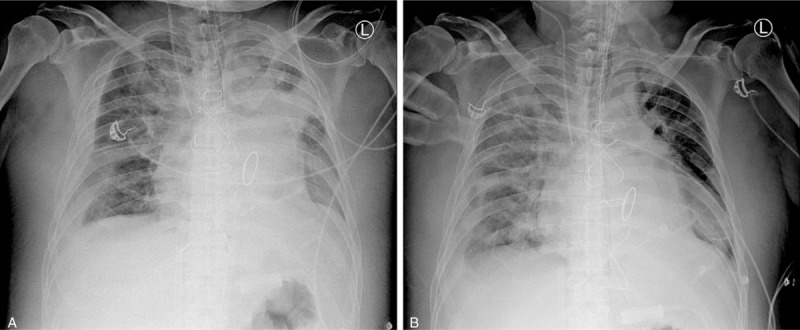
A, Chest radiograph after sodium polystyrene sulfonate aspiration exhibited a new consolidation in the left upper lung. B, After bronchoscopic removal of the foreign material, rapid resolution of left upper lung consolidation was observed 4 hours after the aspiration episode.
